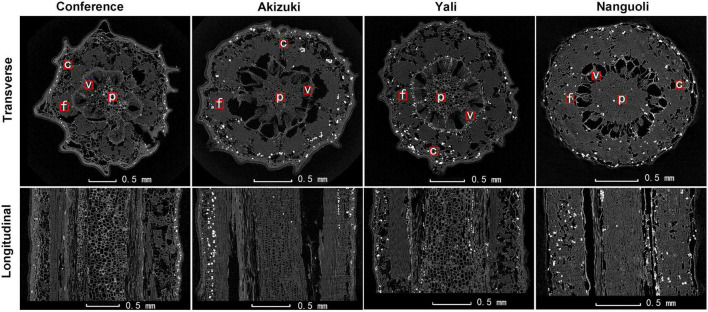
Representative transverse and longitudinal X-ray CT images of the fresh pedicel. Black areas indicate the air-filled tissue, dark gray areas indicate plant tissue, and white spots indicate water-filled tissue. f, fiber cap; p, pith; v, vessels; c, cortex.
